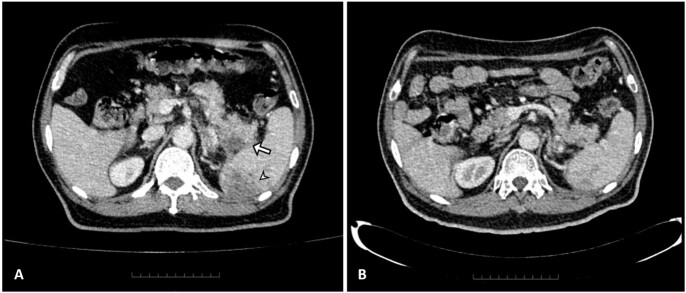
(A) Contrast-enhanced computed tomography image showing a heterogenous hypodense lesion at the pancreatic tail (arrow) and multiple ill-defined splenic lesions (arrowhead). (B) Smaller lesions at the pancreatic tail and spleen after 35 days of antibiotics (Case 1).
